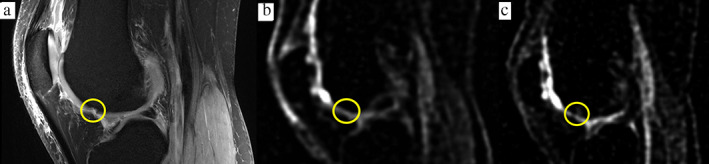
A 39‐year‐old male patient who had a small cartilage defect less than 15% of the cartilage thickness (ICRS grade 2 lesion) in the proximal trochlear region of the lateral femoral condyle. (a) A fat‐suppressed proton density (FS‐PD) image in a sagittal orientation shows a lesion with intra‐chondral signal alterations. Sodium ^23^Na‐MRI images generated (b) 3 days and (c) 4 weeks after the defect.
